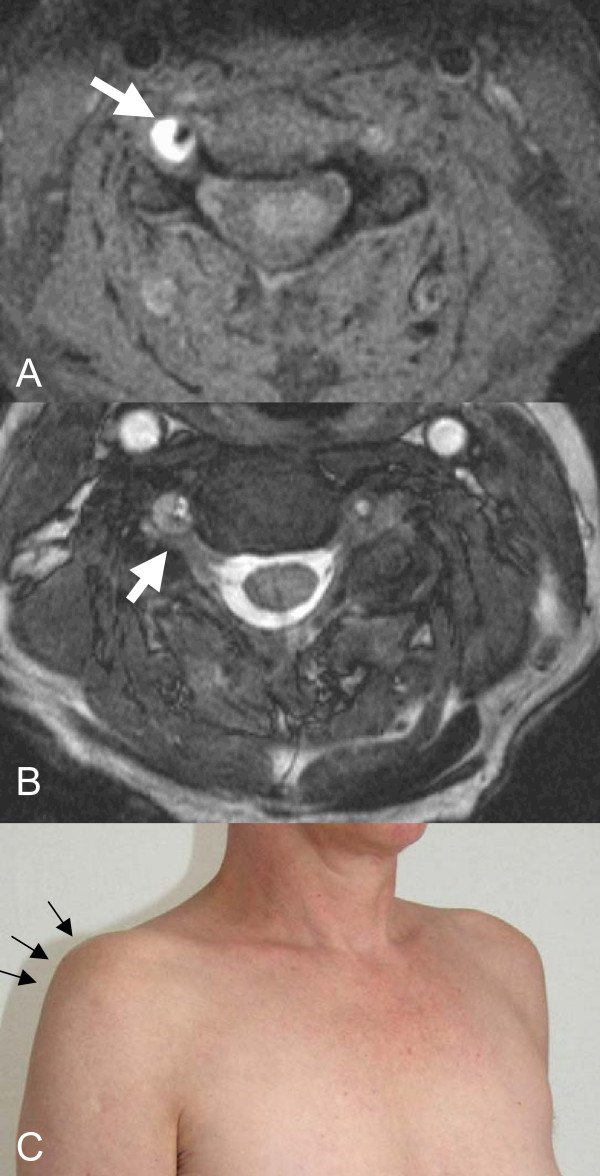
MRI of the cervical spine, axial views (A,B): The T1-weighted fat-saturated axial image (A) shows an eccentric hyperintensity surrounding the right VA at C5 level (arrow) indicating an intramural hematoma. The T2-weighted image (B) indicates compression of spinal root C5 (arrow) by the dissecting aneurysm.
